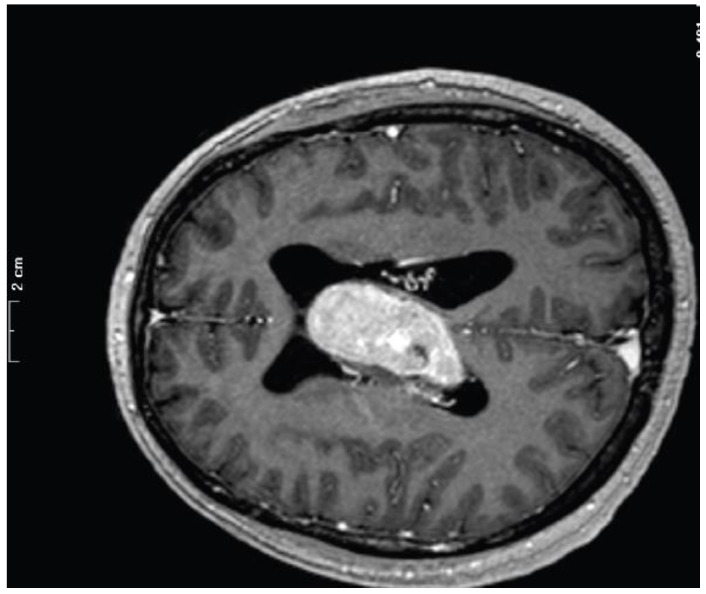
Magnetic resonance imaging of case 23M, showing IVM within the third and lateral ventricles.
